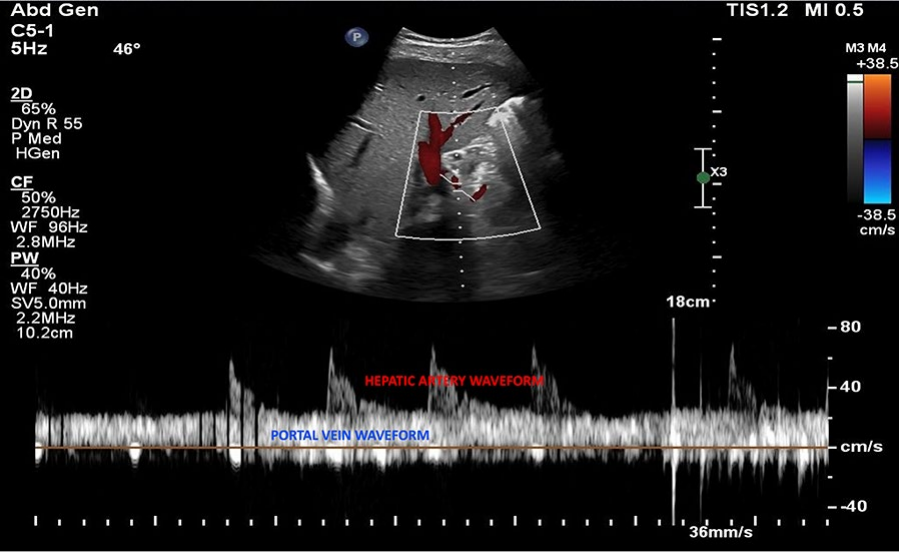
Pulsed-wave Doppler tracing of the PV with hepatic arterial flow interference. PV, portal vein
